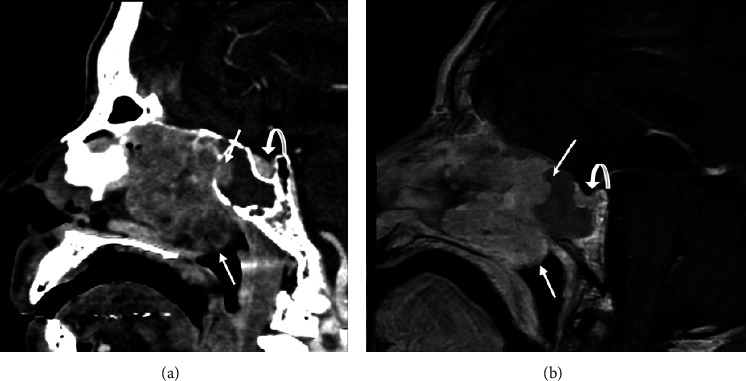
Sagittal views: postcontrast images of (a) CT facial bones and (b) MRI facial bone T1-weighted sequence which reveal the craniocaudal extent of a left nasal cavity heterogeneously enhancing mass with mild protrusion of the posterior border into the sphenoid sinus and superior nasopharynx (solid arrows). The pituitary gland (curved arrows) can also be seen with a similar enhancement pattern to that of the left nasal cavity mass.
